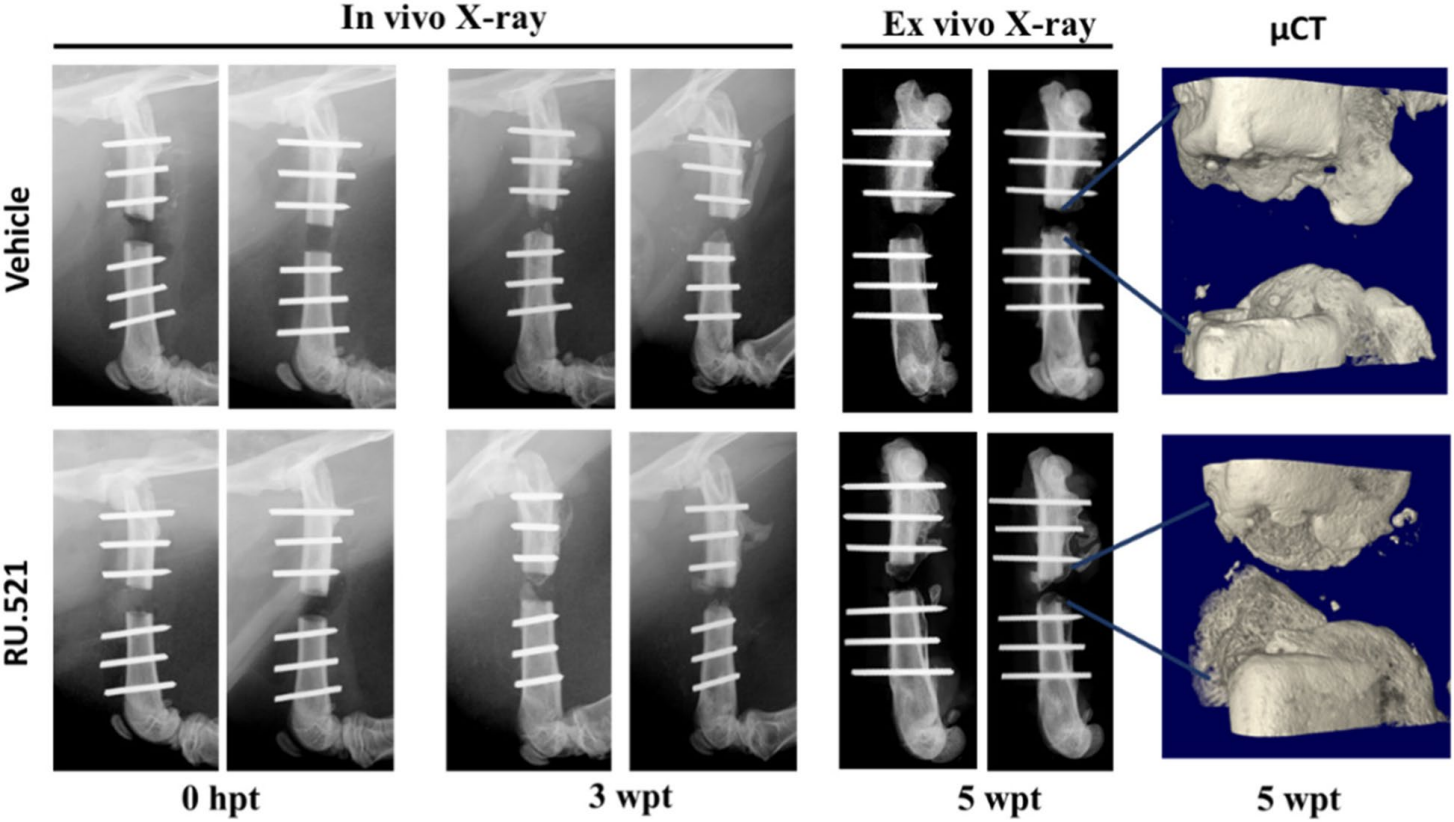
Radiographic and micro-computed tomography images of the fractured callus at 0 h post-trauma (hpt), 3 weeks post-trauma (wpt) and 5 wpt. Two representative images of vehicle (*n* = 5) and RU.521 (*n* = 8) are shown
